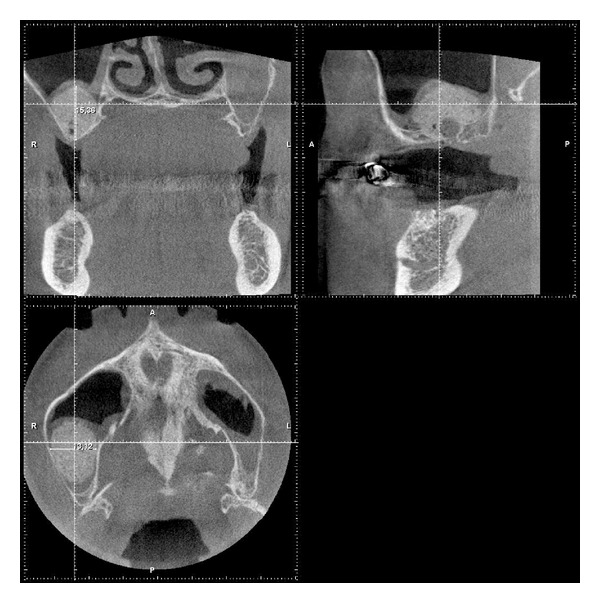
Case 2: CBCT scan 7 months after tHUCSL-Intralift: the completion of the calcification process except some smaller patches of undermineralized areas can be observed.
